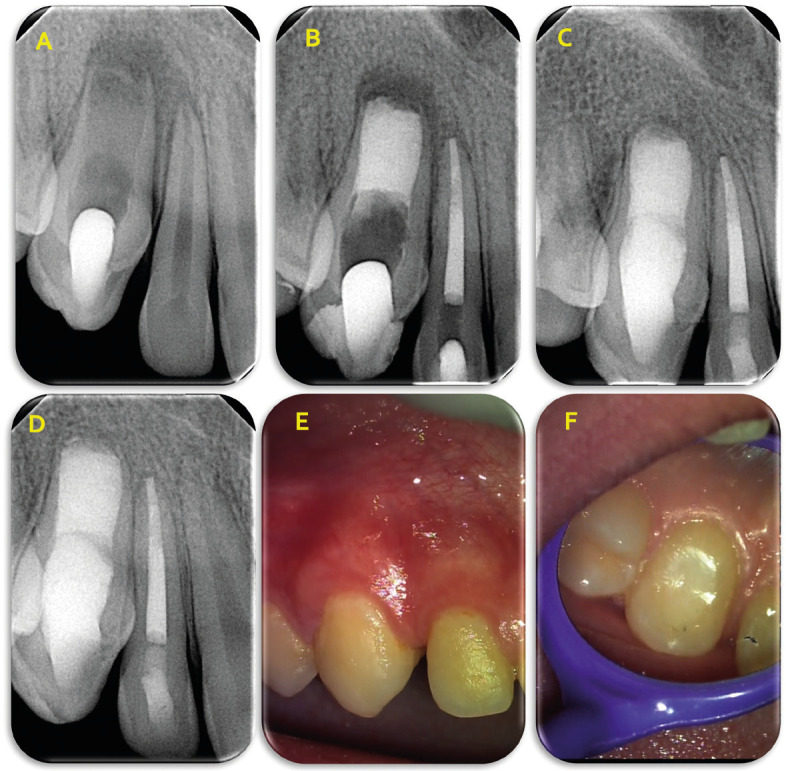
A. Periapical radiograph confirming the complete removal of the invagination, B. Final radiograph of the case, C. 1st follow-up radiograph at 12 months D. 2nd follow-up radiograph at 24 months, E,F. Clinical appearance of the teeth and the soft tissues during second recall examination.
